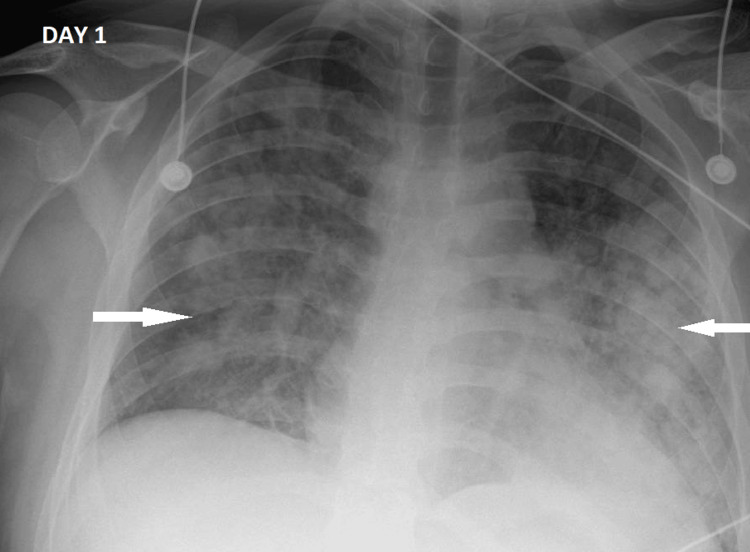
Anteroposterior chest radiograph obtained on day 1 (day of admission) Demonstrating bilateral, diffuse pulmonary infiltrates (white arrows) consistent with acute respiratory failure.
